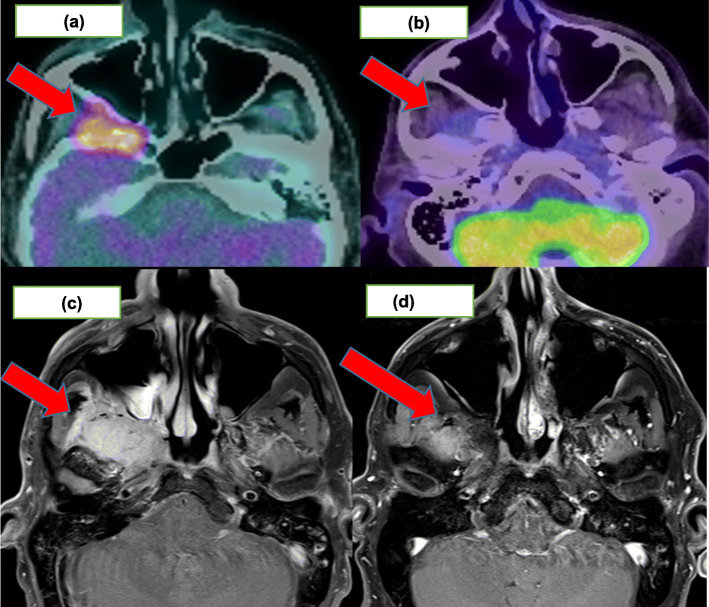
68 year old man with imaging for advanced cutaneous squamous cell carcinoma involving the right skull base, right infratemporal fossa and right trigeminal nerve managed with cemiplimab 350 mg/3-weekly. a demonstrating avid disease at baseline and (b) a CMR post 24 cycles. c demonstrating 41 × 23 mm enhancing mass on baseline MRI and (d) an ongoing RECIST1.1 PR post 24 cycles
